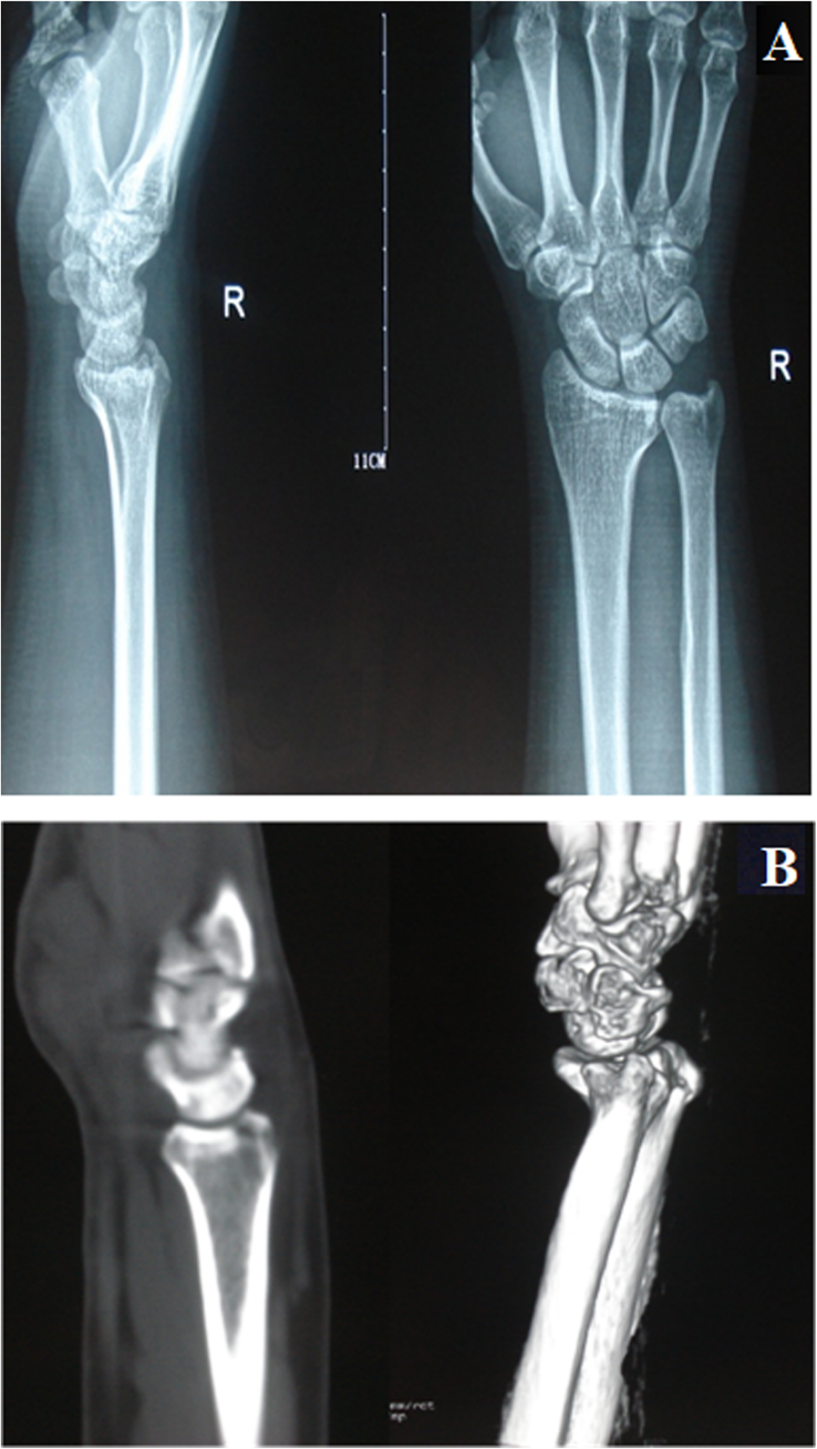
PA X-ray (a) and CT scan (b) of dorsal single-column DRDPF
